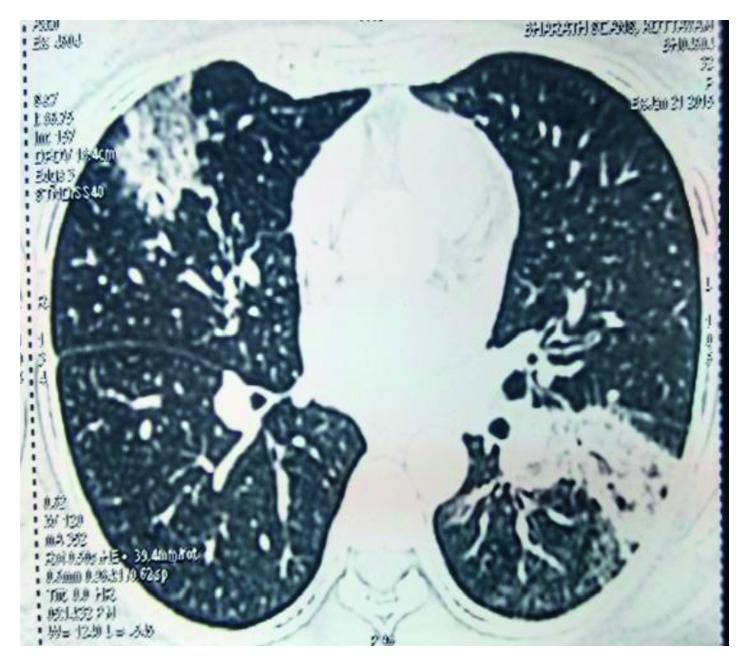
HRCT chest showing peripherally based patchy, subsegmental lesions of the upper lobe and left lower lobe.
